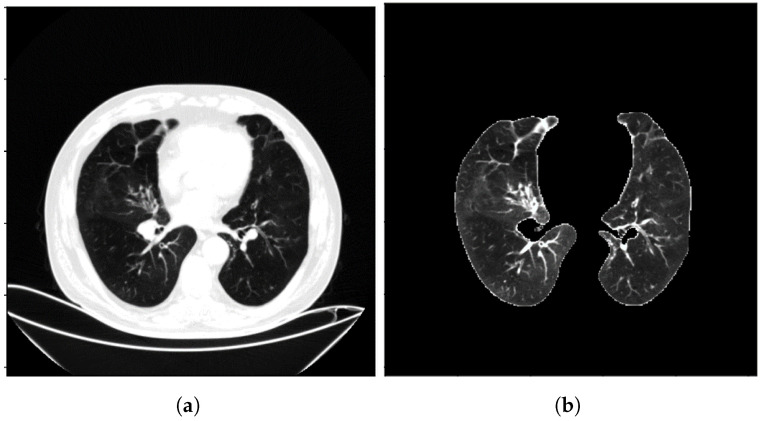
The CT image data before and after preprocessing (larger scale), (a) is before preprocessing and (b) is after preprocessing.
